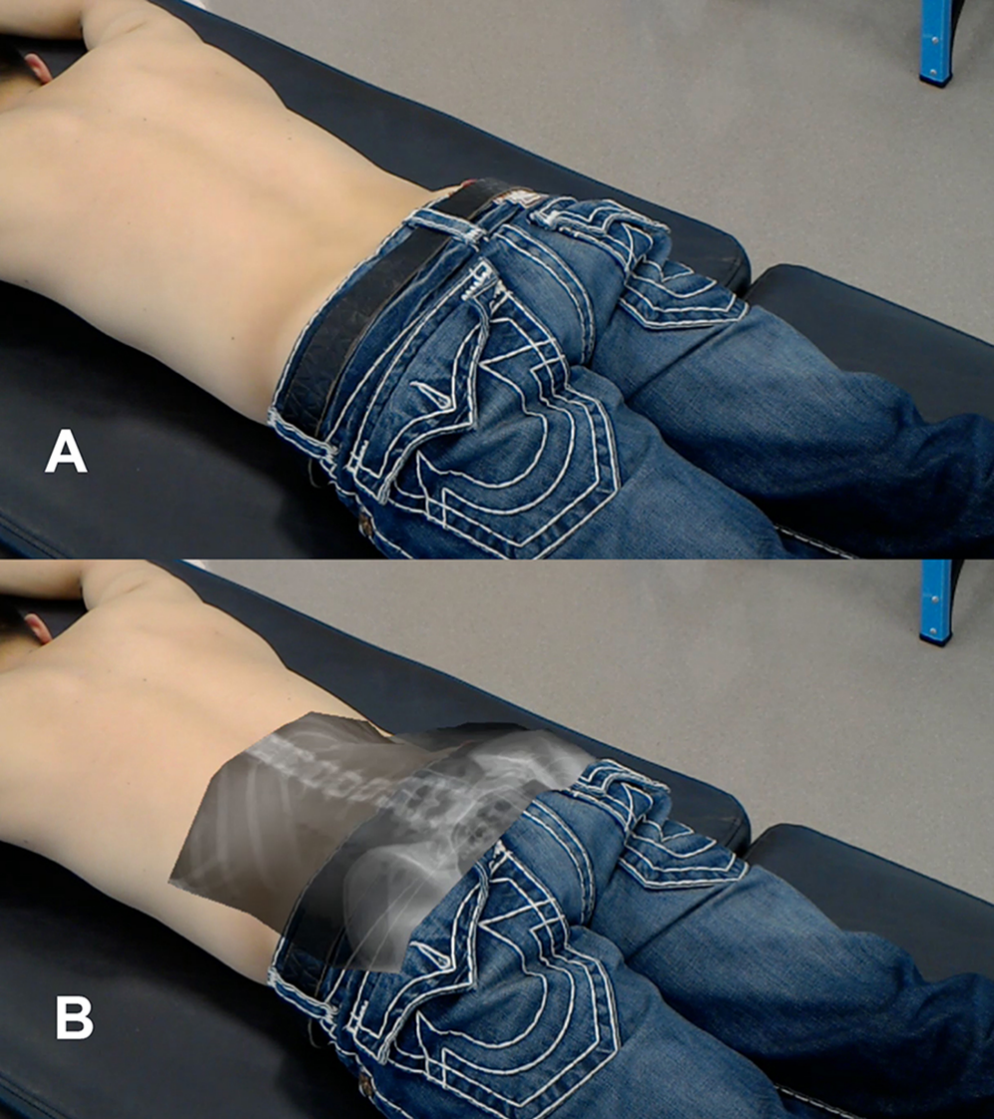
Examiner view of the participant (A) without OST-HMD and (B) with OST-HMD display displaying radiograph superimposed on a participant.
